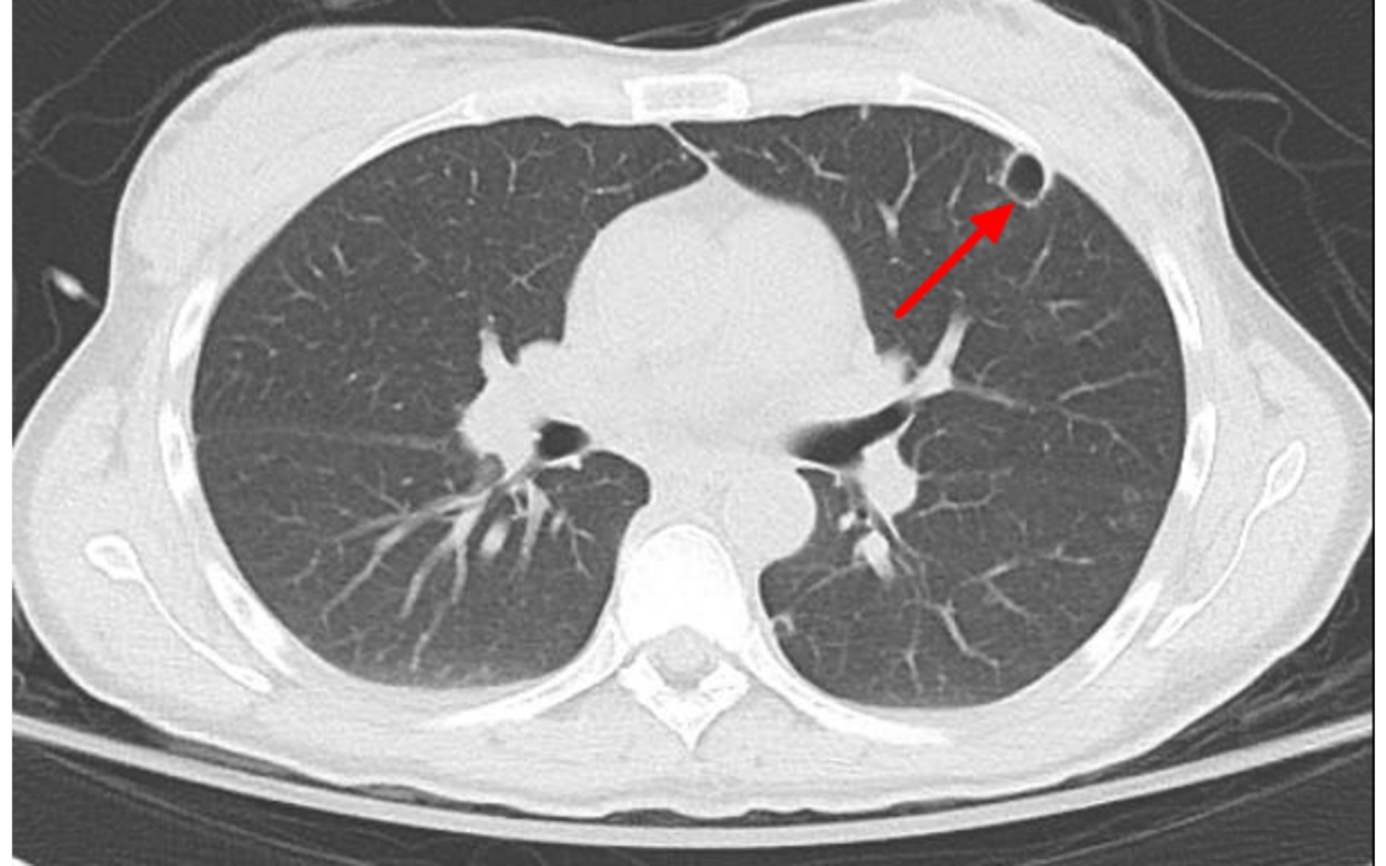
Non-contrast CT chest showing a left cavitary lesion (red arrow) on the left lung.
